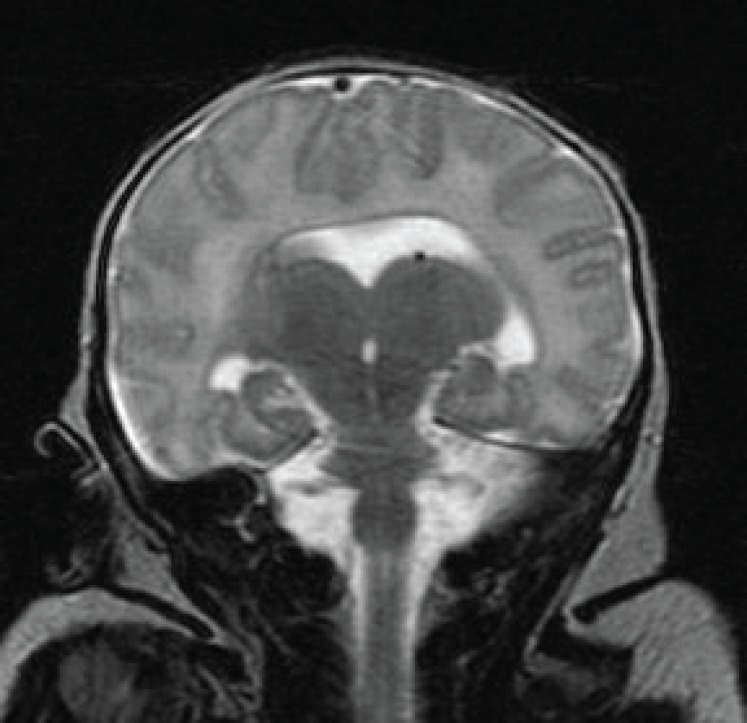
Coronal T2 weighted MRI of the brain showing fused frontal lobes of both the cerebral hemisphere
